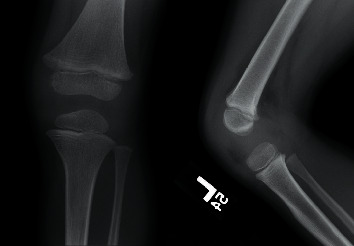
AP and lateral radiographic view of the left knee joint demonstrating joint effusion without presence of fracture.
